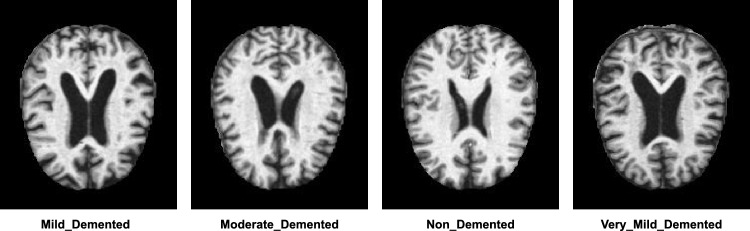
Sample MRI Images of Alzheimer’s disease based on four different cases: Mild-demented, Moderate-demented, Non-demented and Very-mild-demented.
